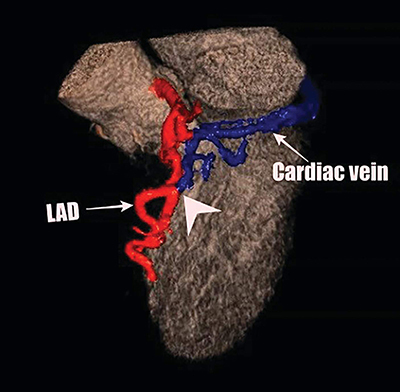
The volume-rendered 3D reconstruction and magnified image from the coronary CT angiogram show the fistulous connection (white arrowhead) between the dilated left anterior descending coronary artery (red vascular) and the great cardiac vein (blue vascular) much more demonstratively.
